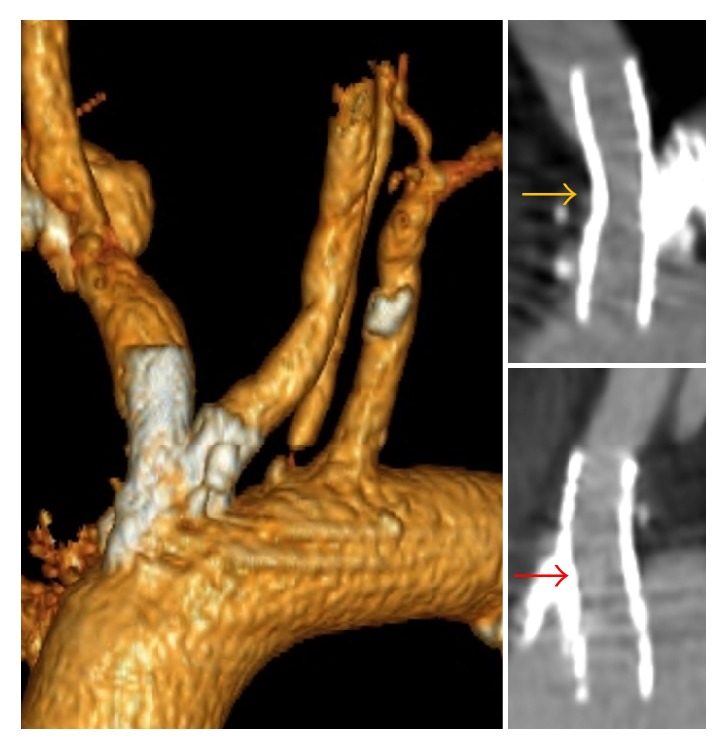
3-month CT angiogram demonstrates excellent dilatation of the innominate (yellow arrow) and left common carotid artery (red arrow) in the supra-aortic portion.
